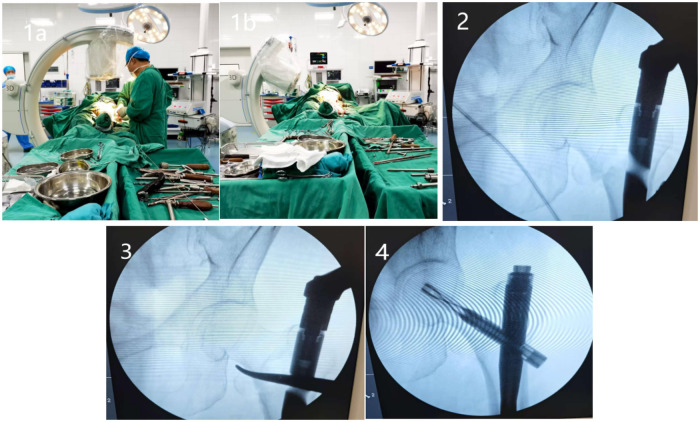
Case 1: (1a,b) Intraoperatively, the lateral beam of the C-arm fluoroscope was aligned with the lesser trochanter, and the operating table was tilted to the right. This approach allowed for the acquisition of a near-lateral radiograph. Upon completion of the procedure, a routine frog-leg position x-ray was obtained, which confirmed that the spiral blade was properly seated within the femoral neck. (2–4) Following satisfactory manual reduction, mild displacement of the proximal fracture fragment was observed during intramedullary nail insertion. An auxiliary anterior hip incision was then utilized to achieve effective re-reduction, after which internal fixation and locking were performed.
